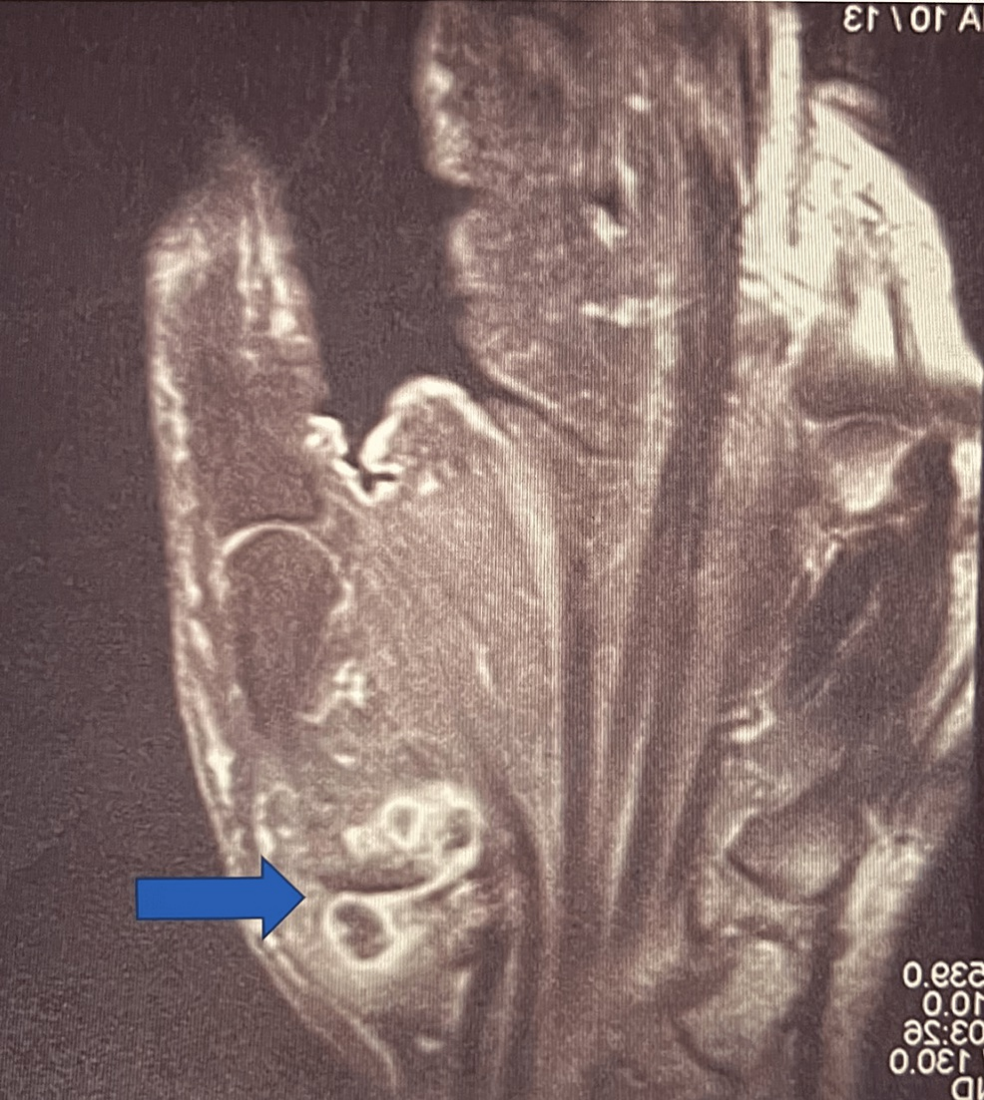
MRI of a right first carpometacarpal joint ganglion of a 60-year-old patient. The multiloculated complex ganglion is marked by the blue arrow.
